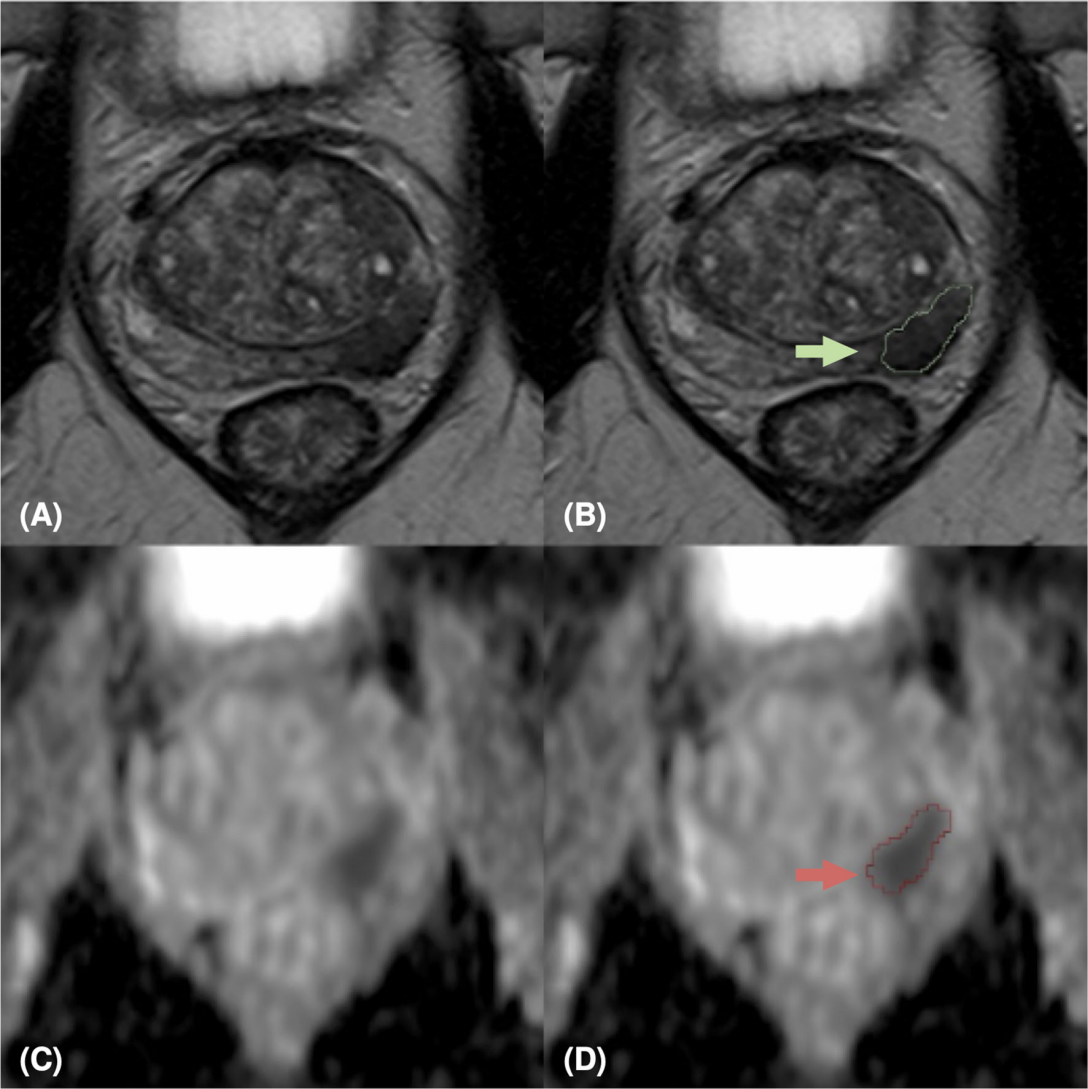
MpMRI of a 69-years old patient with positive mpMRI result (PI-RADS=5), PSA level=7 ng/ml, GS=4+4 (ISUP 4). MRI zoomed images containing the target lesion, respectively axial T2-weighted image (A), ADC map (C), and their relative lesion segmentations (B, D). The green (B) and red (D) arrows point out the segmented lesion in T2 and ADC images, respectively.
